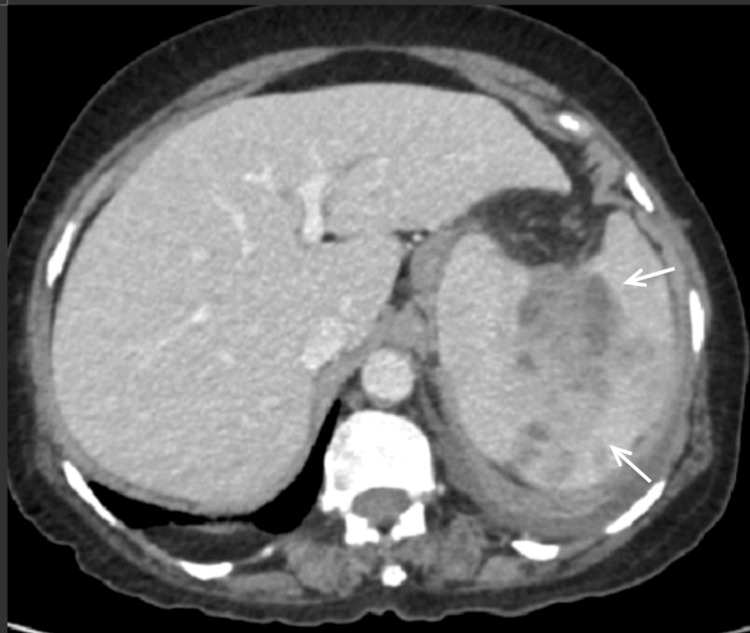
CECT abdomen showing multiple irregular peripherally enhancing multi locular collections within the splenic parenchyma CECT: Contrast-Enhanced Computed Tomography.
